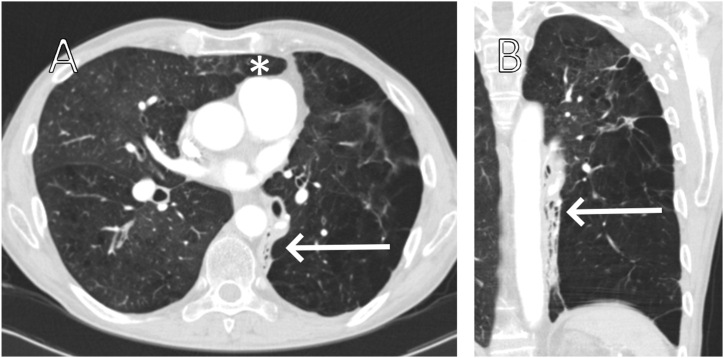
Contrast-enhanced chest CT-scan with pulmonary windowing with axial (A) and coronal (B) reconstructions. This demosntrates left lower lobe (LLL) collapse, with the lobe being retracted along the spine (A, B, arrow). Right lung herniation can be seen (A, star), with the posterior limit of the lung lying against the anterior aspect of the pulmonary trunk.
